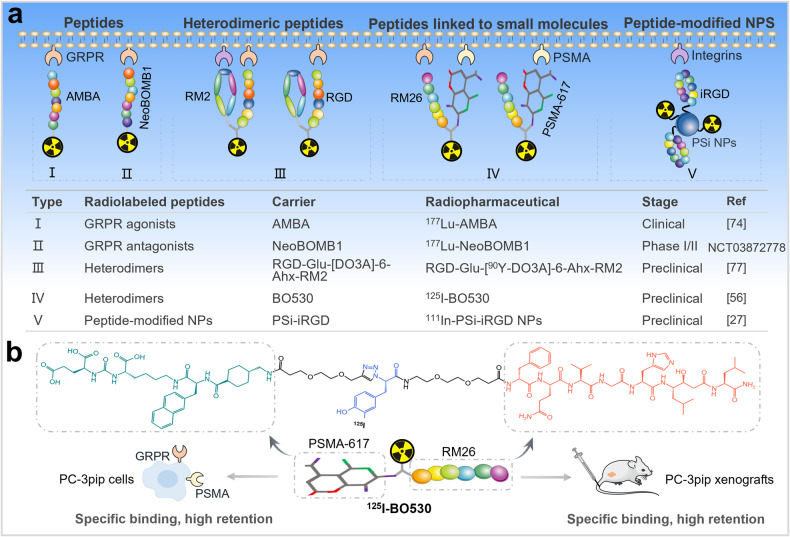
Radiolabeled peptides in prostate cancer therapy. a Radiolabeled peptides, heterodimeric peptides, heterodimers of peptides linked to small molecules, and peptide-modified NPs for prostate cancer therapy. b Radiolabeled GRPR/PSMA heterodimers for prostate cancer therapy.
